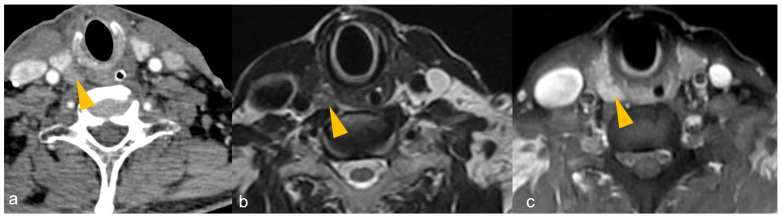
(a–c): CT and MRI of parathyroid adenoma in a patient with primary hyperparathyroidism. (a) Late venous phase 4DCT shows a well defined oval-shaped hypodense lesion (arrowhead) measuring 0.9 × 0.8 cm posterior to the inferior pole of right lobe of thyroid gland, suggestive of parathyroid adenoma. T2WI (b) shows intermediate signal intensity of the parathyroid adenoma, which shows intense post-contrast enhancement (arrowhead in (c)).
